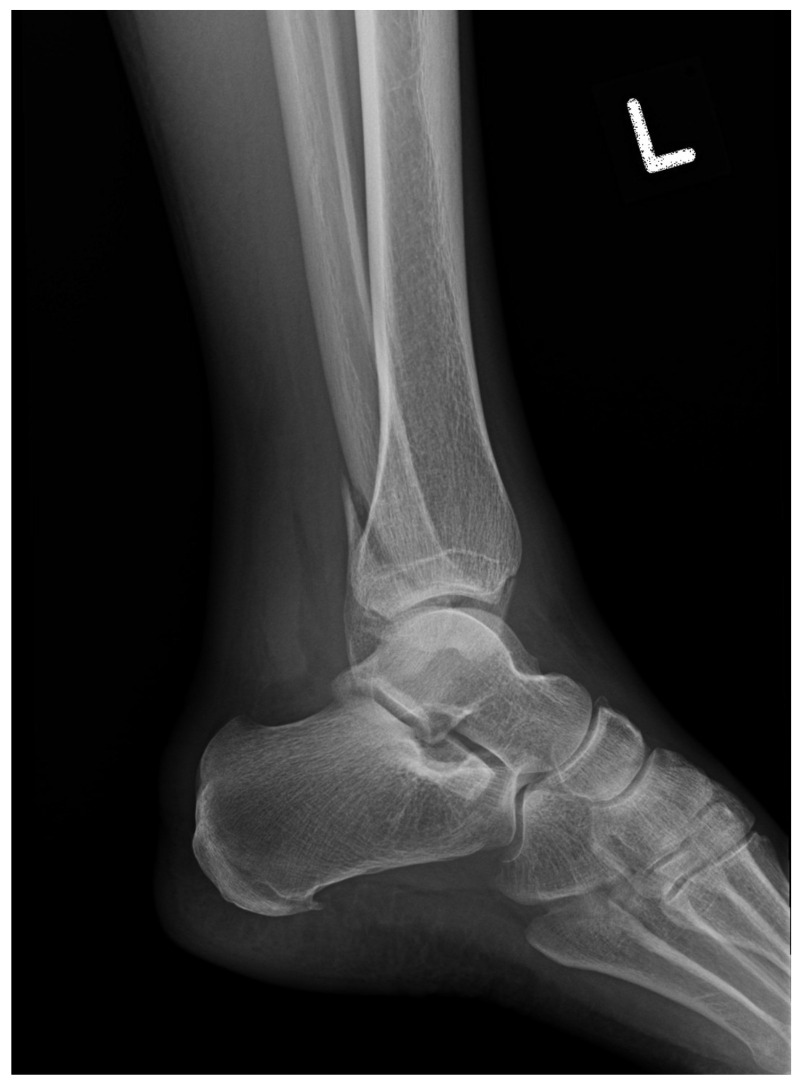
Distal fibular fracture as seen on lateral ankle radiograph.
